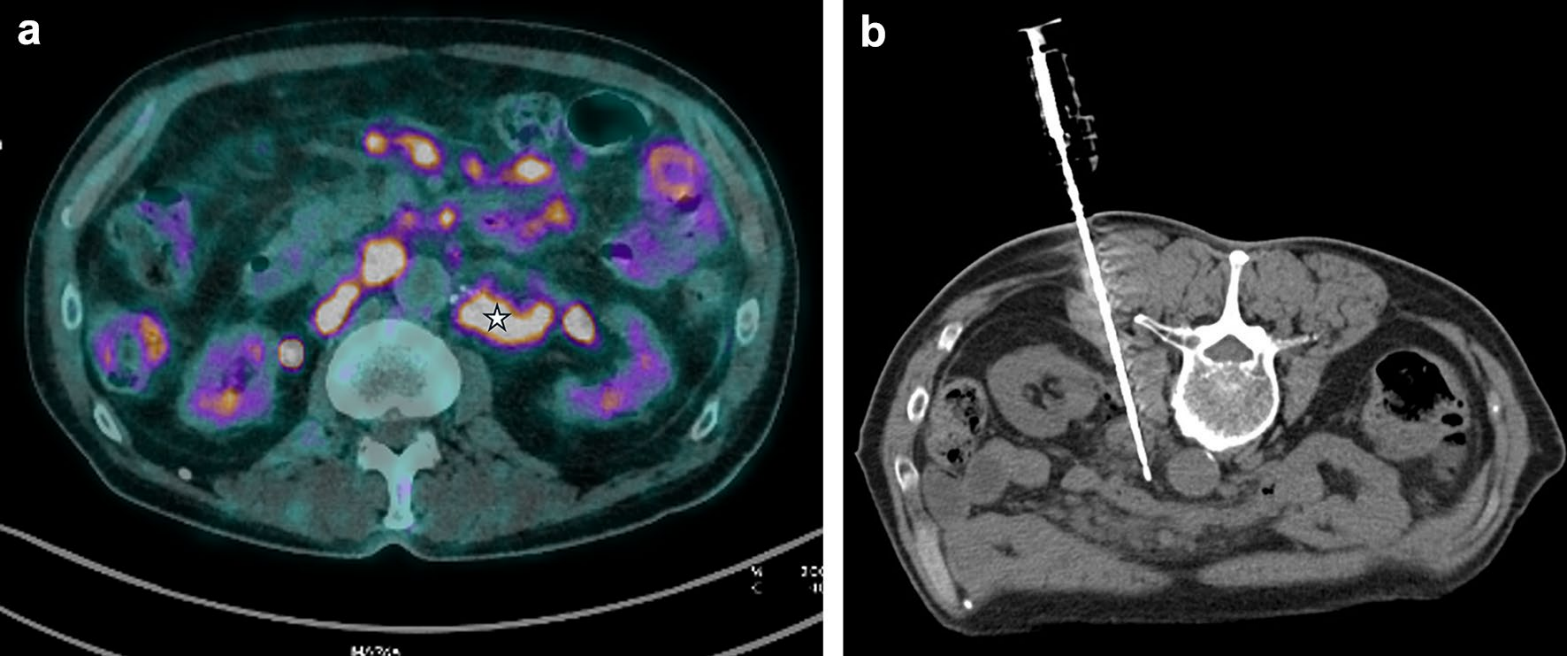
Core needle biopsy of enlarged retroperitoneal lymph nodes in a patient with Whipple’s disease. Preoperative [18F]FDG PET/CT (A) image shows multiple enlarged lymph nodes in the retroperitoneal space with a maximum SUV of 12.6. Left para-aortic lymph nodes are chosen as the safest biopsy target (star). With the patient lying in a prone position a CT-guided translumbar coaxial biopsy was performed: image (B) shows multiplanar reformation of the needle trajectory
